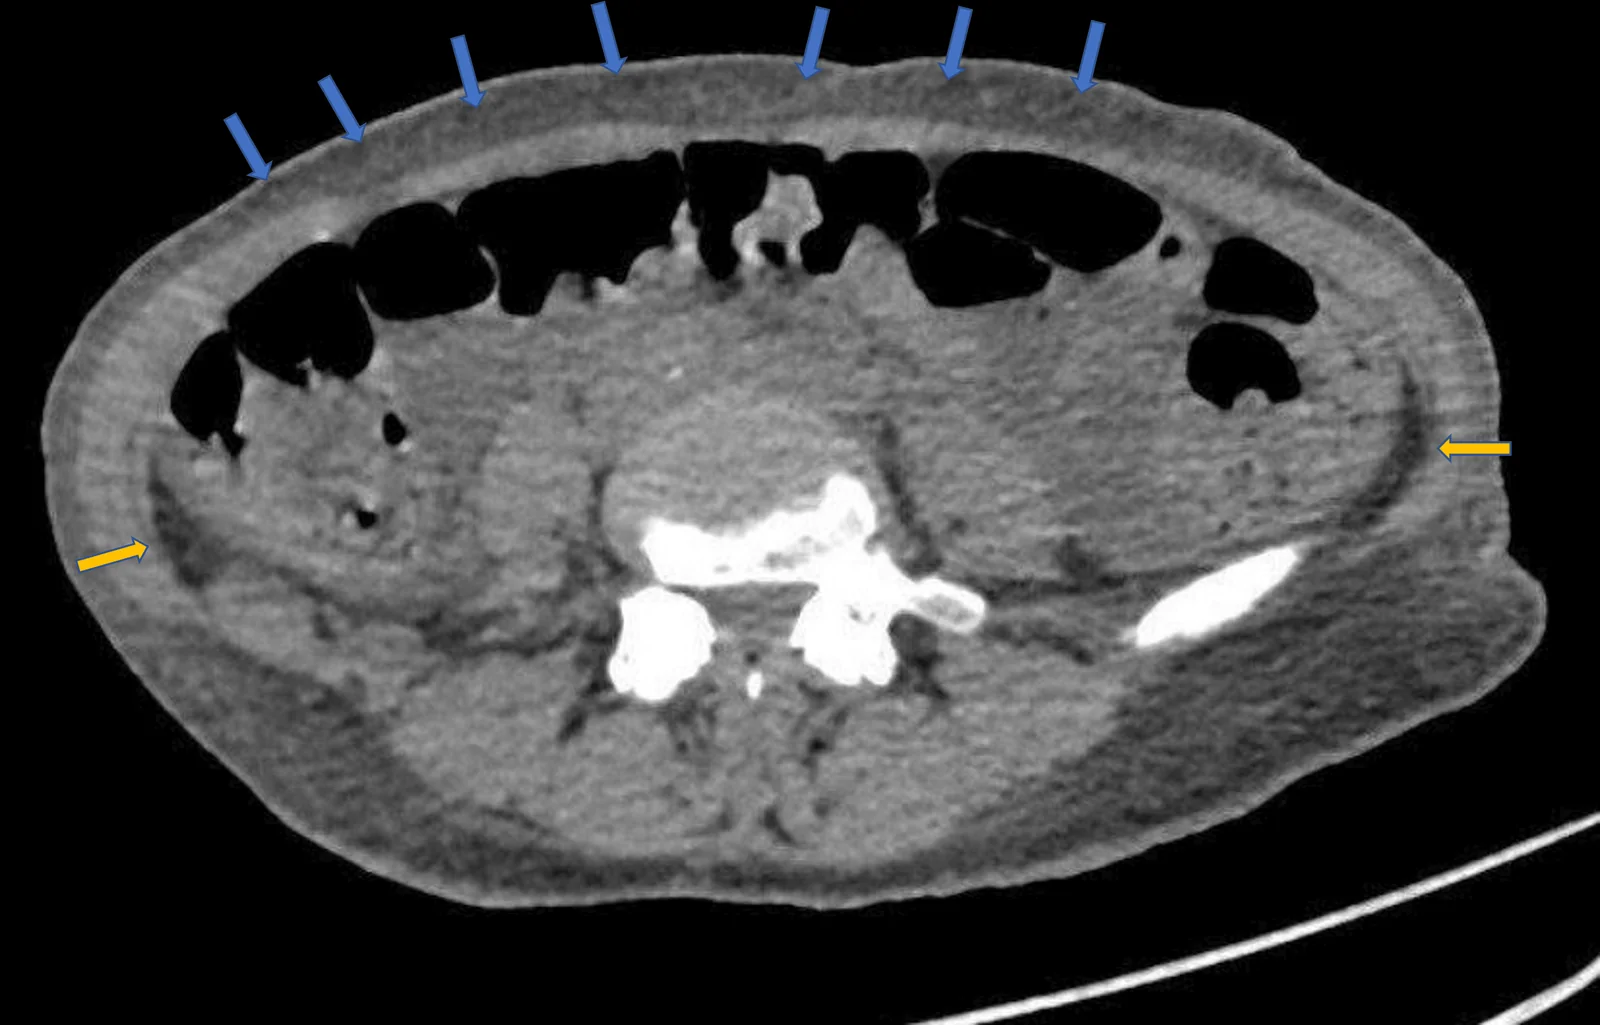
Axial non-contrast CT abdomen and pelvis. Blue arrows: diffuse subcutaneous oedematous changes, consistent with plasma leakage. Orange arrows: mild free intra-abdominal fluid. Kidneys normal; no pneumoperitoneum or obstruction.
